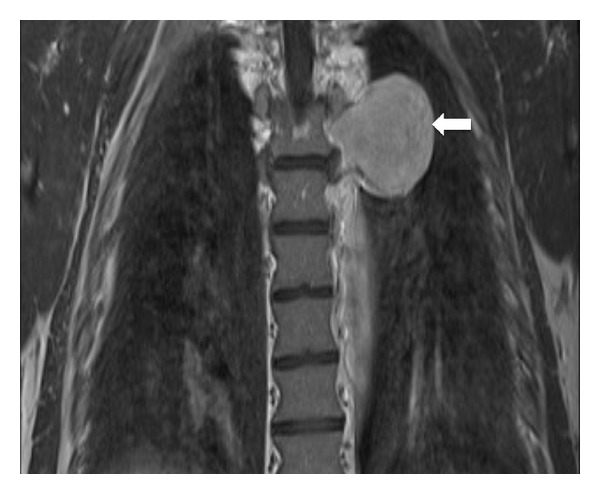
Chest MRI (coronal T1 gradient echo sequence with intravenous gadolinium contrast enhancement) in a 50-year-old male patient with Castleman disease. The left paraspinal pleural-based mass demonstrates homogenous enhancement following intravenous gadolinium administration (arrow).
